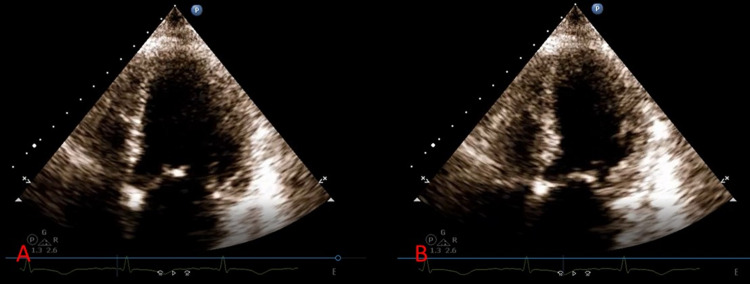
Apical four-chamber view. (A) Left ventricular end-diastole and (B) left ventricular end-systole. Severe hypokinesis of the apex and mid left ventricular segments with preservation of the basal segments.
